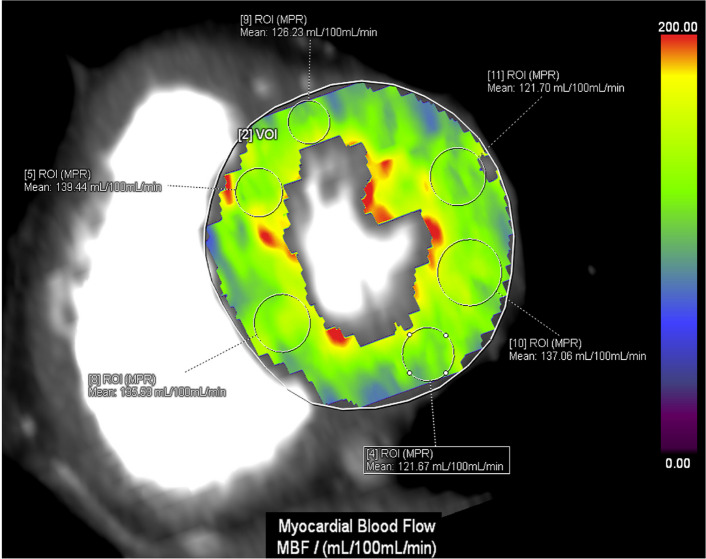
Quantitative assessment of myocardial blood flow (MBF) using dynamic stress computed tomography perfusion (CTP). Short-axis MBF map obtained from dynamic stress CTP, with segmental regions of interest (ROIs) placed according to the American Heart Association 17-segment model
